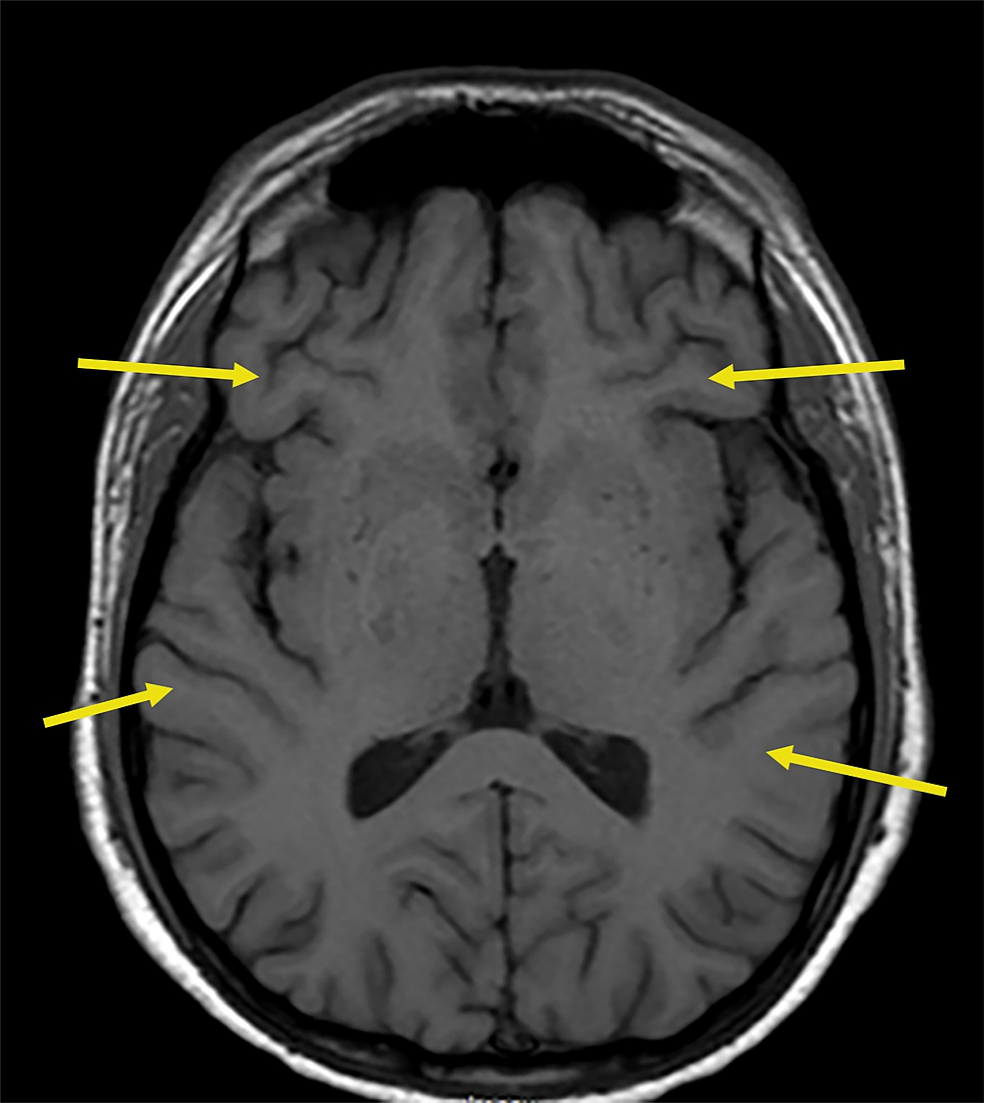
Brain MRI showing sulcal prominence (arrows) MRI: magnetic resonance imaging
